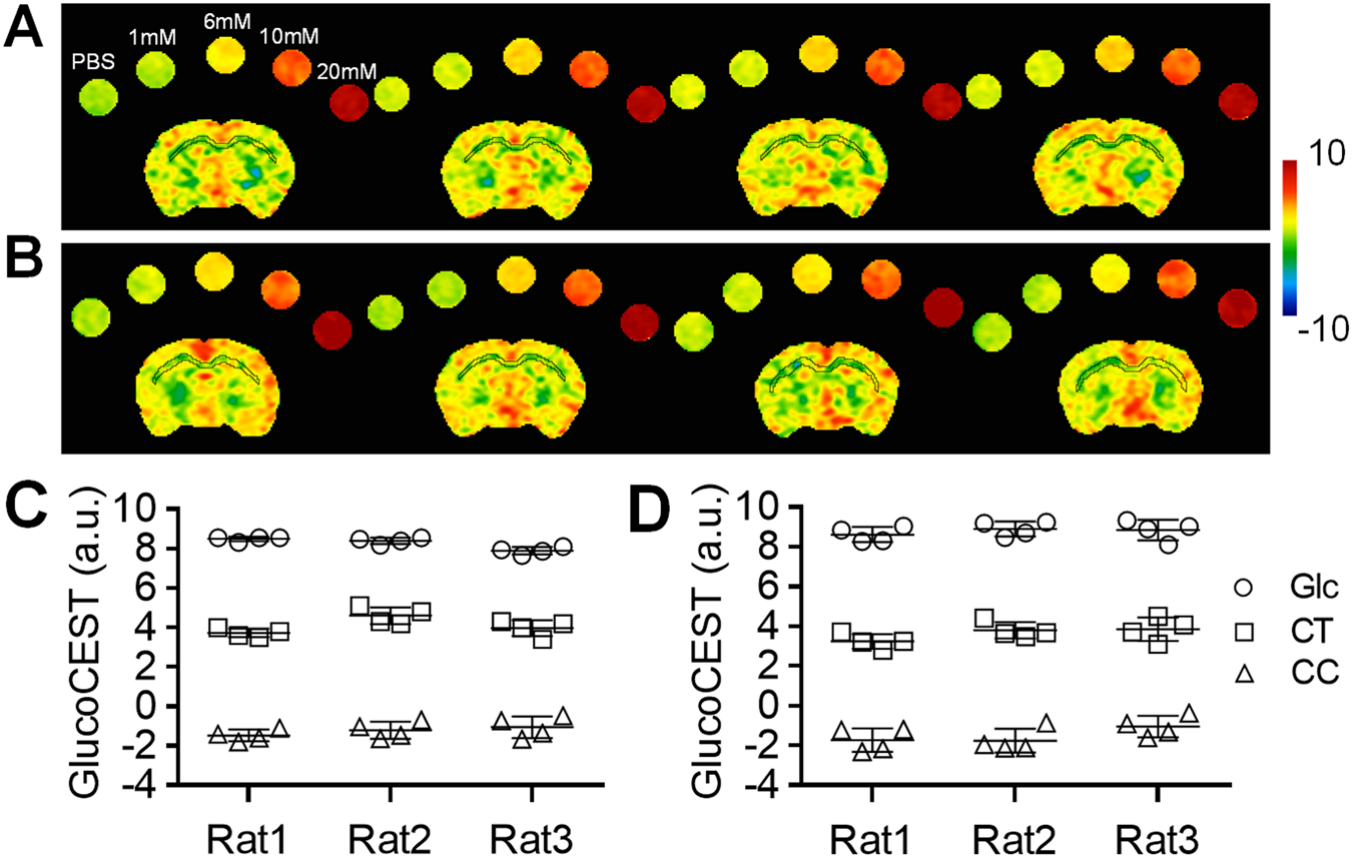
Representative glucoCEST-weighted images of repeating scans display consistent contrast in the brain from four continuous scans (A) and four weekly scans (B). The ROI quantification of the cortex shows an intrasubject coefficient of variation 8.4 ± 2.2% in the continuous scans (C) and 15.1 ± 2.9% in the weekly scans (D) (n = 3). CT: cortex, CC, corpus callosum, Glc: glucose, PBS: phosphate-buffered saline.
